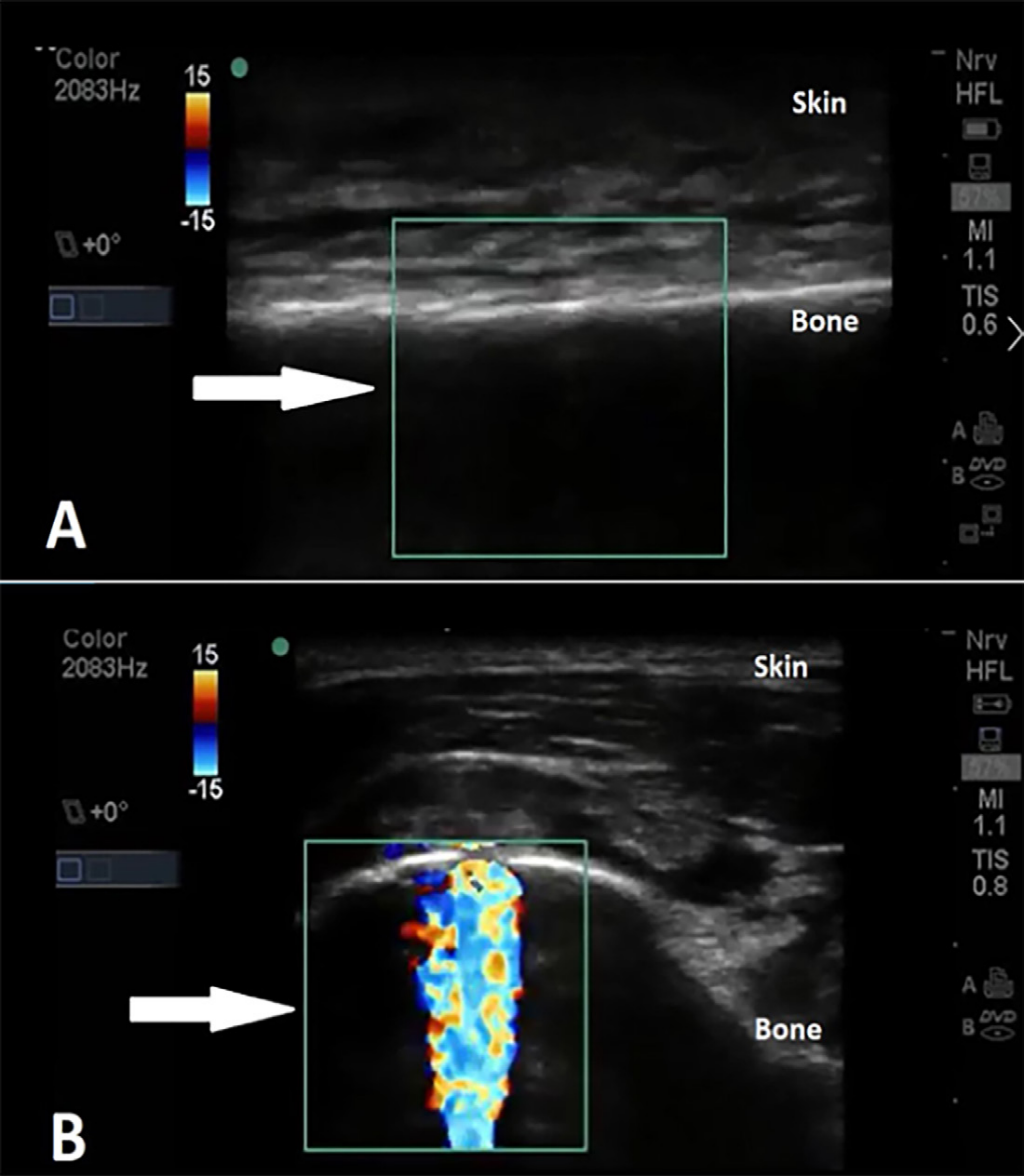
A) Ultrasound image of non-functioning left proximal tibia intraosseous needle demonstrating no flow (arrow). B) Ultrasound image of properly functioning right humerus intraosseous needle demonstrating flow (arrow).
